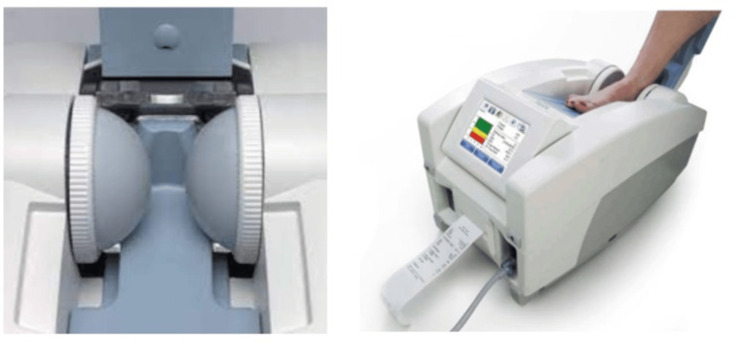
Inflated membranes used to transmit ultrasound waves.
